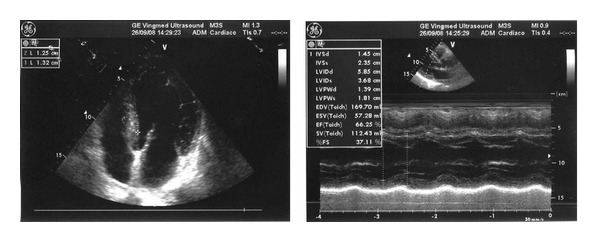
Case 2: echocardiography before the last bortezomib cycle.
